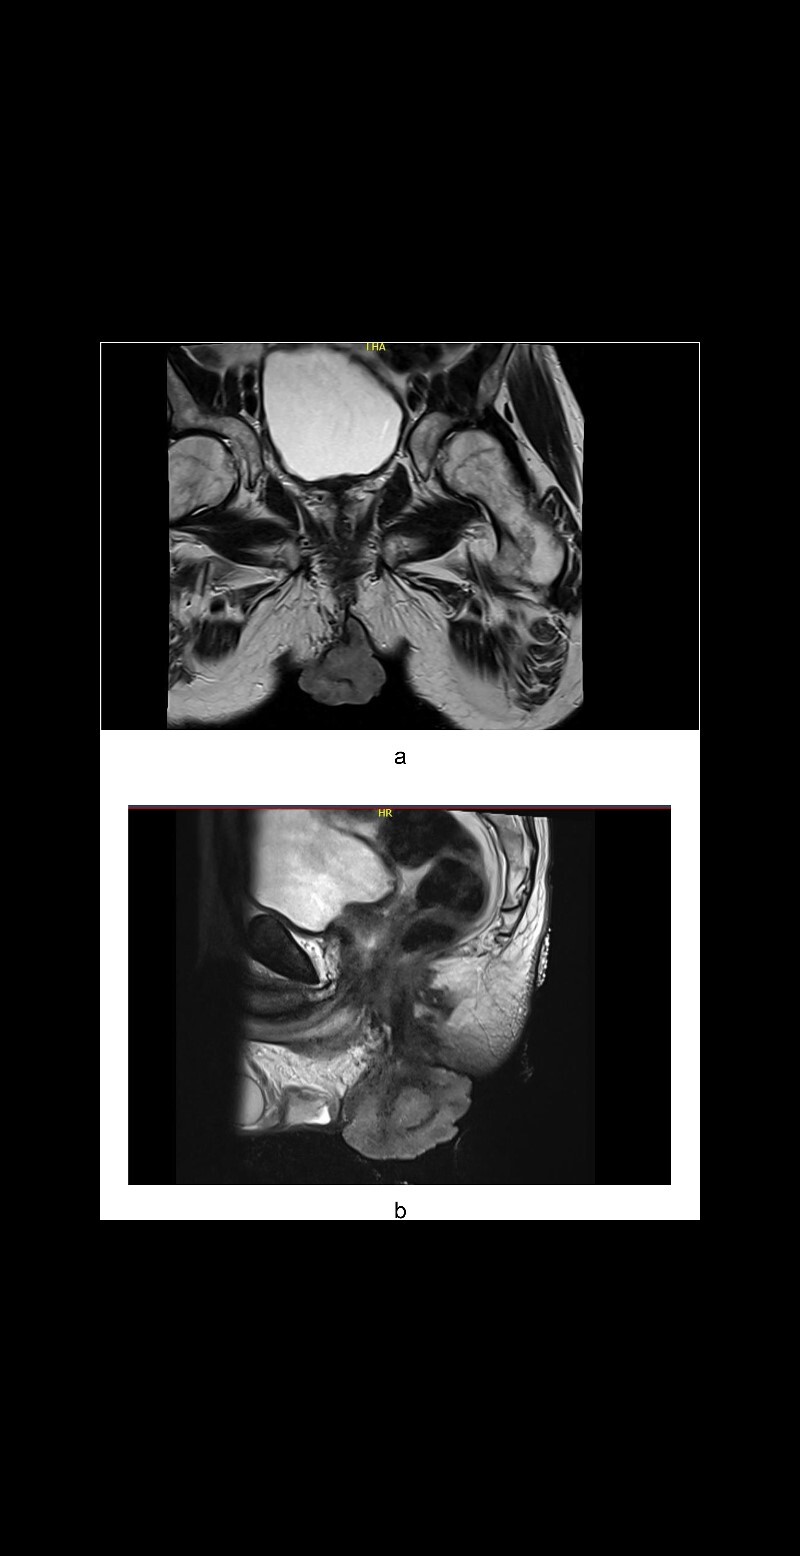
(a) Axial plane MRI pelvis: A large perianal mass. (b) Sagittal plane MRI pelvis: A large perianal mass
